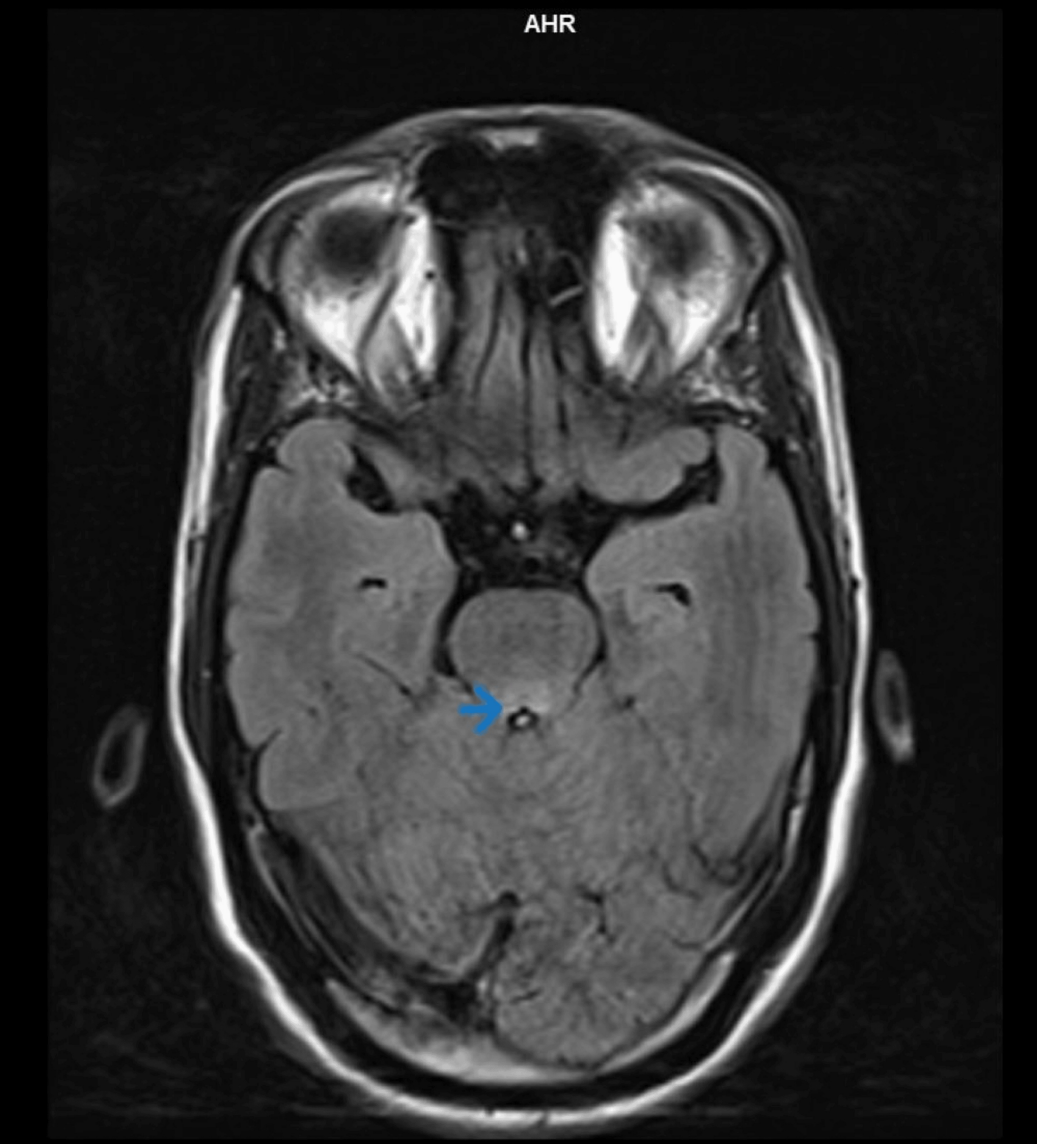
T2 FLAIR hyperintensities involving dorsal pons (arrow) on the axial section at the level of mid-pons FLAIR: fluid-attenuated inversion recovery
